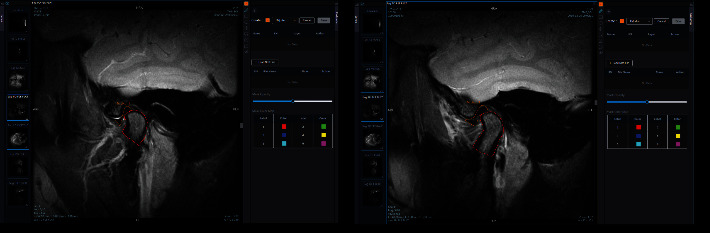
MRI images showing the tracings of TMJ disc and condyle in a radiomic platform.
